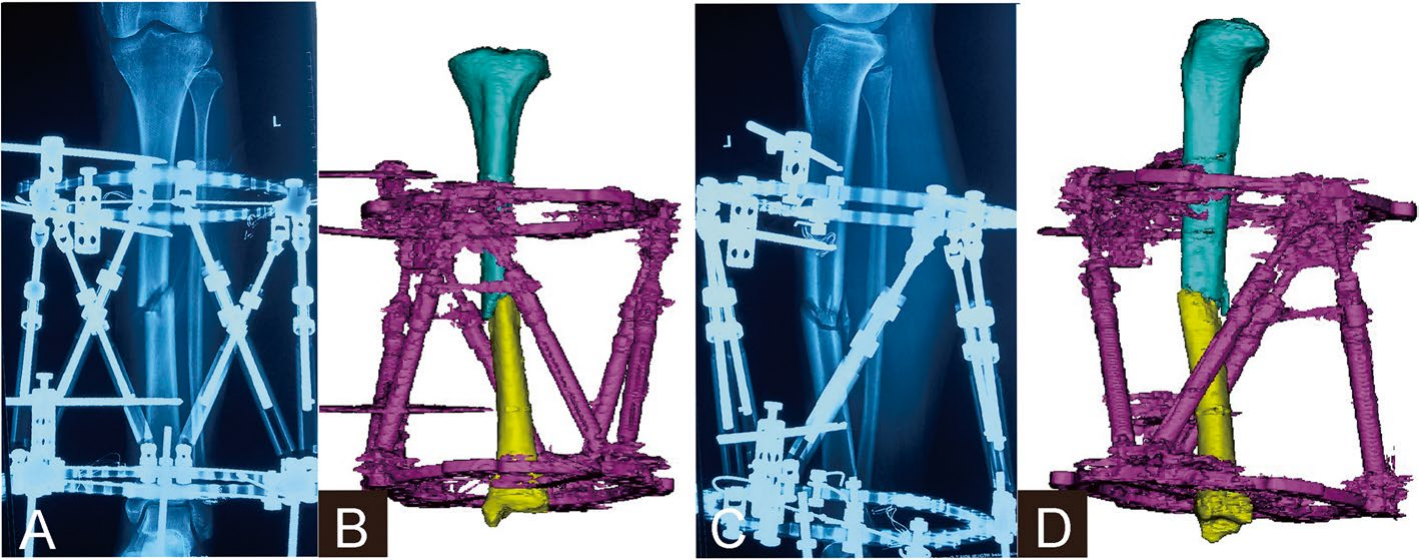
A 41-year-old man with traumatic multidimensional displacement in tibia treated by the hexapod external fixator, and underwent staged correction trajectory. a and b Immediate postoperative X-rays and three-dimensional reconstruction in the AP view. c and d Immediate postoperative X-rays and three-dimensional reconstruction in the lateral view
